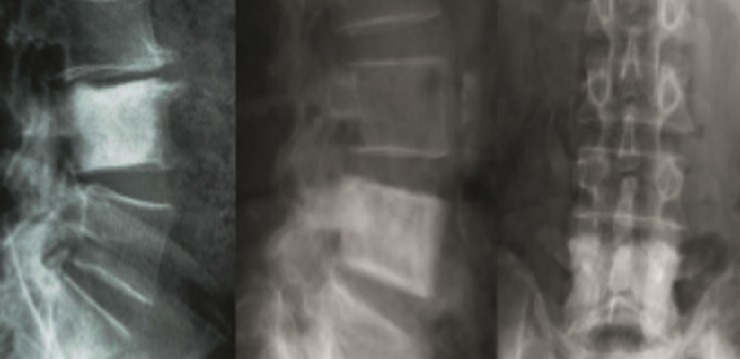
Radiographie standard face et profil du rachis lombaire objectivant une vertèbre L4 en ivoire (hyperdense)
